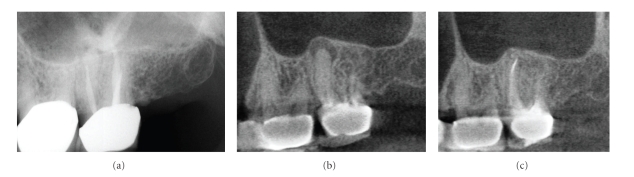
A periapical radiograph of the maxillary left first molar (a) shows an area of low density surrounding the mesial root with incomplete endodontic treatment. A contemporaneous CBCT parasagittal image (b) shows a chronic periradicular osteoperiostitis, or “halo lesion,” where the apical periodontitis has caused displacement of the periosteum but did not penetrate the antral floor. Three months after retreatment, CBCT imaging (c) demonstrates complete apical resolution.
